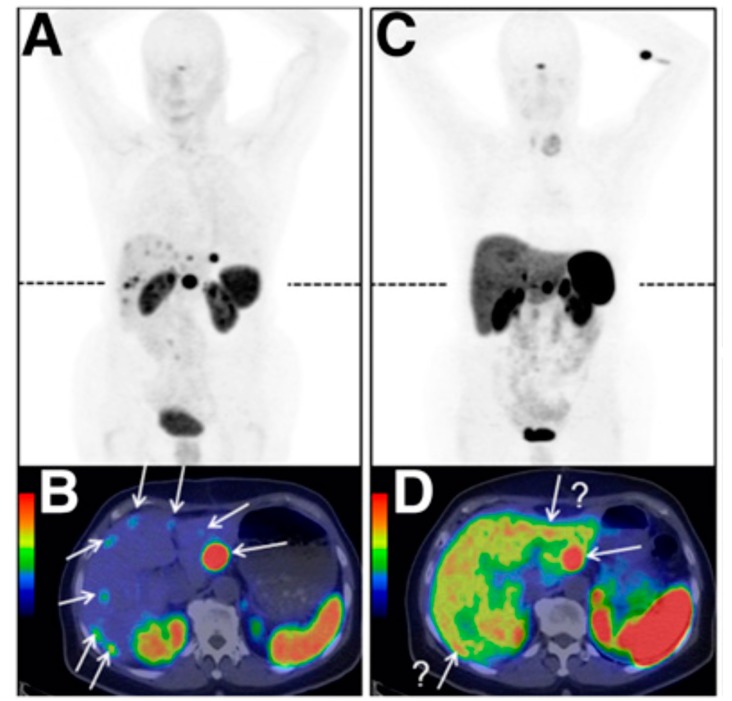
[^68^Ga]Ga-OPS202 PET/CT [antagonist—(A,B)] and [^68^Ga]Ga-DOTATOC PET/CT [agonist—(C,D)] images of a patient with ileal neuroendocrine tumours , showing bilobar liver metastases (dashed lines indicate level of transaxial slices). Studies were performed on the same scanner within 2 months and show same gray (maximal-intensity projections, (A,C) and colour scale (transaxial fusion images, (B,D). Importantly, background activity was lower in liver, intestine, and thyroid with [^68^Ga]Ga-OPS202 than with [^68^Ga]Ga-DOTATOC allowing better identification of the lesions in the liver which are confirmed by subsequent MRI. (Adapted from Nicolas et al., originally puplished in J Nucl Med [142]).
